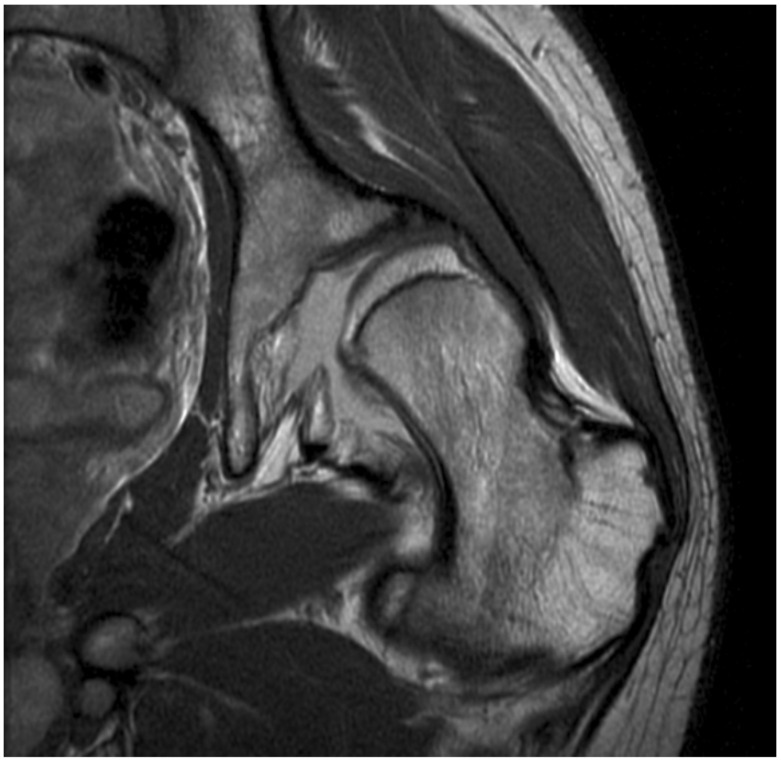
Radiographic features of Stage 4 MSP at the hip: MRI scan of a 14-year old boy, Type IV, left hemiplegia, chronic neglected hip displacement, GMFCS III. Note the full thickness loss of articular cartilage on the lateral aspect of the femoral head. The left hip was successfully reconstructed, but this is salvage surgery. The lost cartilage does not regenerate, and the hip is destined for premature arthrosis and arthroplasty.
